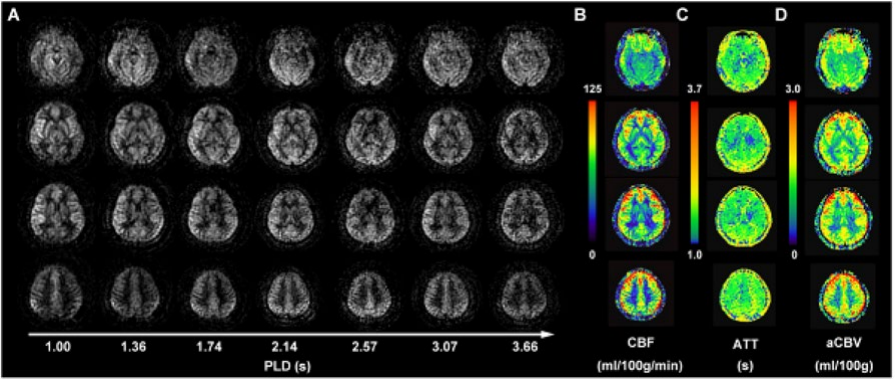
Digram of cerebral perfusion by multi-delay ASL MR imaging. (A) The raw maps of ASL imaging, including different post label delays (PLDs) ranging from 1.00 to 3.66 seconds (s). (B) The arrival-time-corrected cerebral blood flow (CBF) colormaps. (C) The arterial arival time (ATT) colormaps. (D) The arterial cerebral blood colume (aCBV) colormaps
